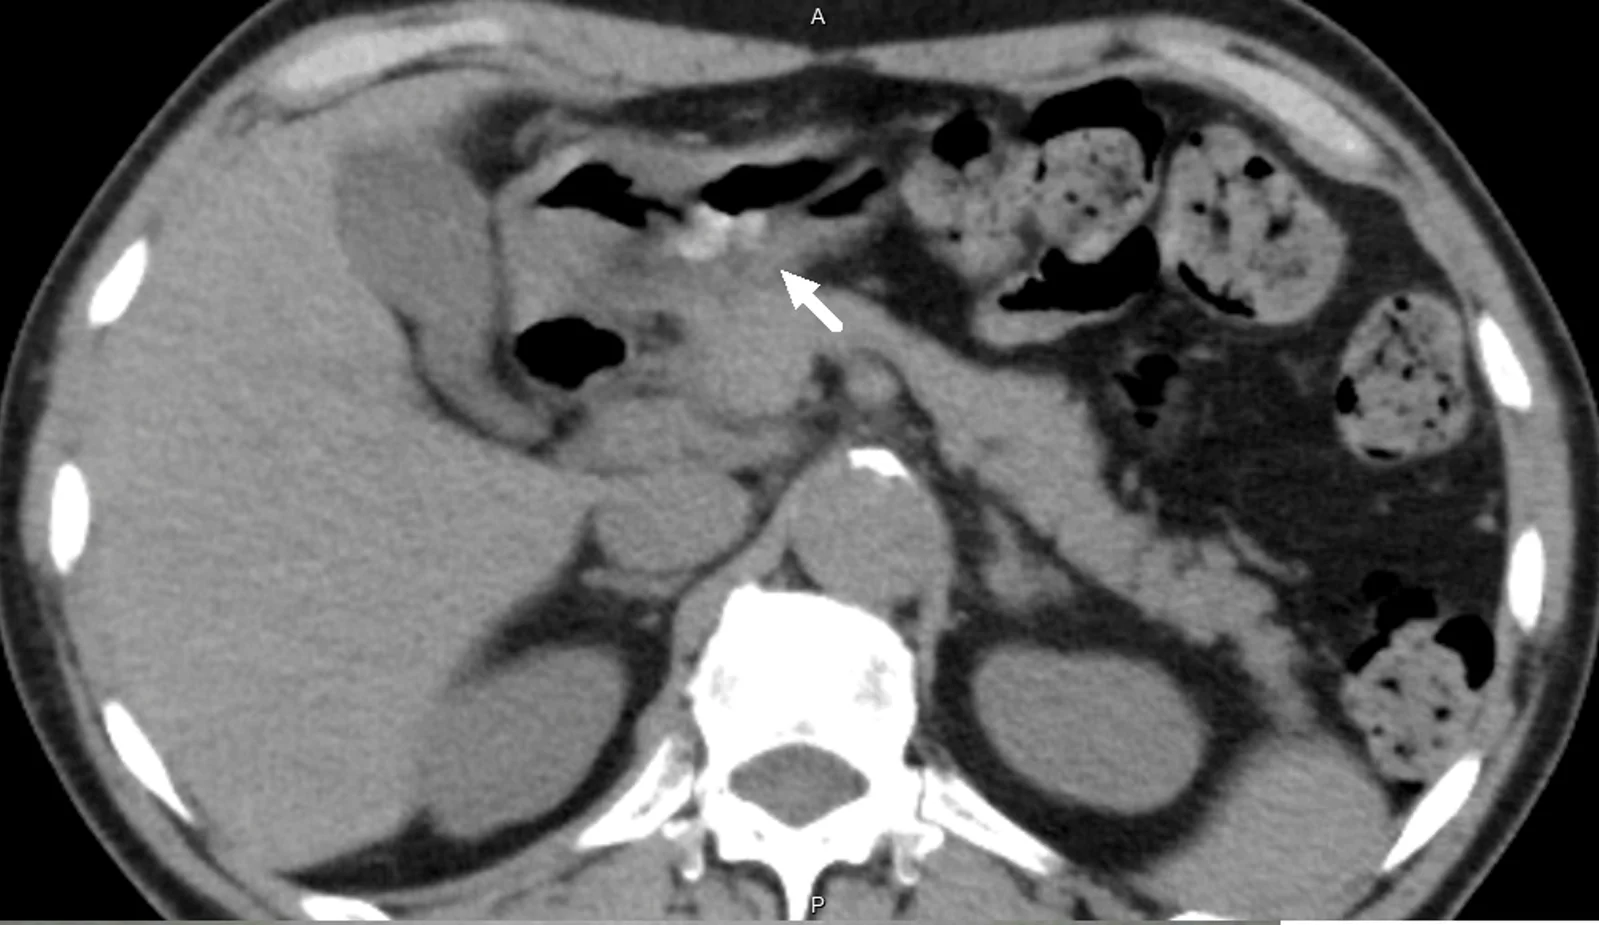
CT Non-contrast chest–abdomen CT showing no definite intraluminal foreign body and no secondary signs of perforation or obstruction. Retrospective radiologic review demonstrated only a subtle low-attenuation structure that could not be confidently distinguished from normal gastric folds or intragastric contents (arrow).
